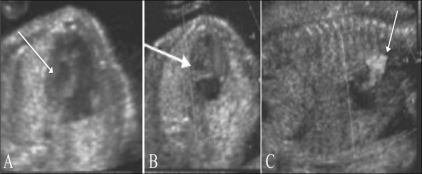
Hypoplastic left heart. USG image (A) shows an echogenic left ventricle (arrow). Doppler images (B,C) show absent flow on the left side (arrow in B), and reversal of flow in the aortic arch (arrow in C)
